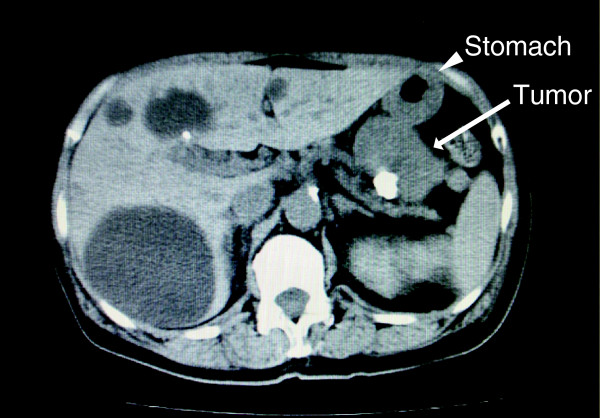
Computed tomographic findings of the tumor. A computed tomographic scan showing a large tumor measuring 56 × 55mm accompanied by calcification. An arrow indicates the tumor; the arrow head indicates the stomach.
